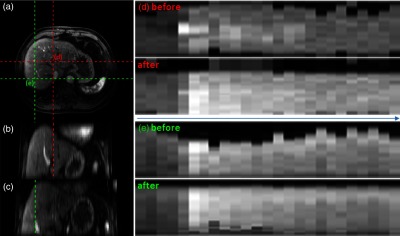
(a) Axial and (b, c) two coronal views for reference volume with (d) red and (e) green dashed line indicating the locations of their corresponding time-cuts for DCE-MRI in the most challenging case #F0002 before and after registration using the GIFTed Demons with random image clustering (rnd-gif). Our method shows considerable improvements in temporal alignment of liver.
